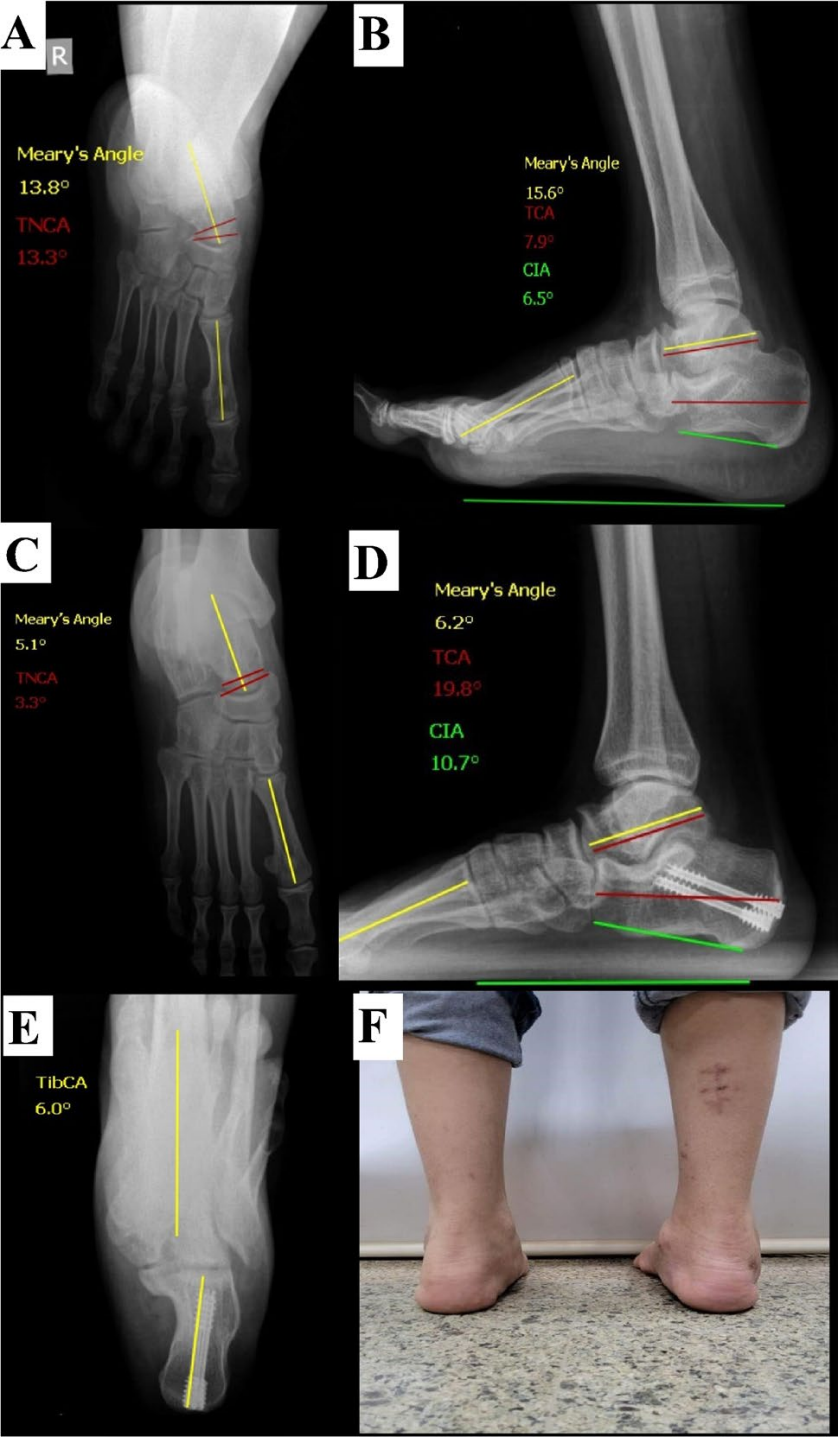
A male patient, 14 years old, had an MDCO procedure. A, B Preoperative radiographs. C–E Last follow-up (18 months) radiographs showing deformity correction (as per angles measured) and restoration of the heel alignment as shown in the axial view. F A clinical image showing restoration of the heel alignment
